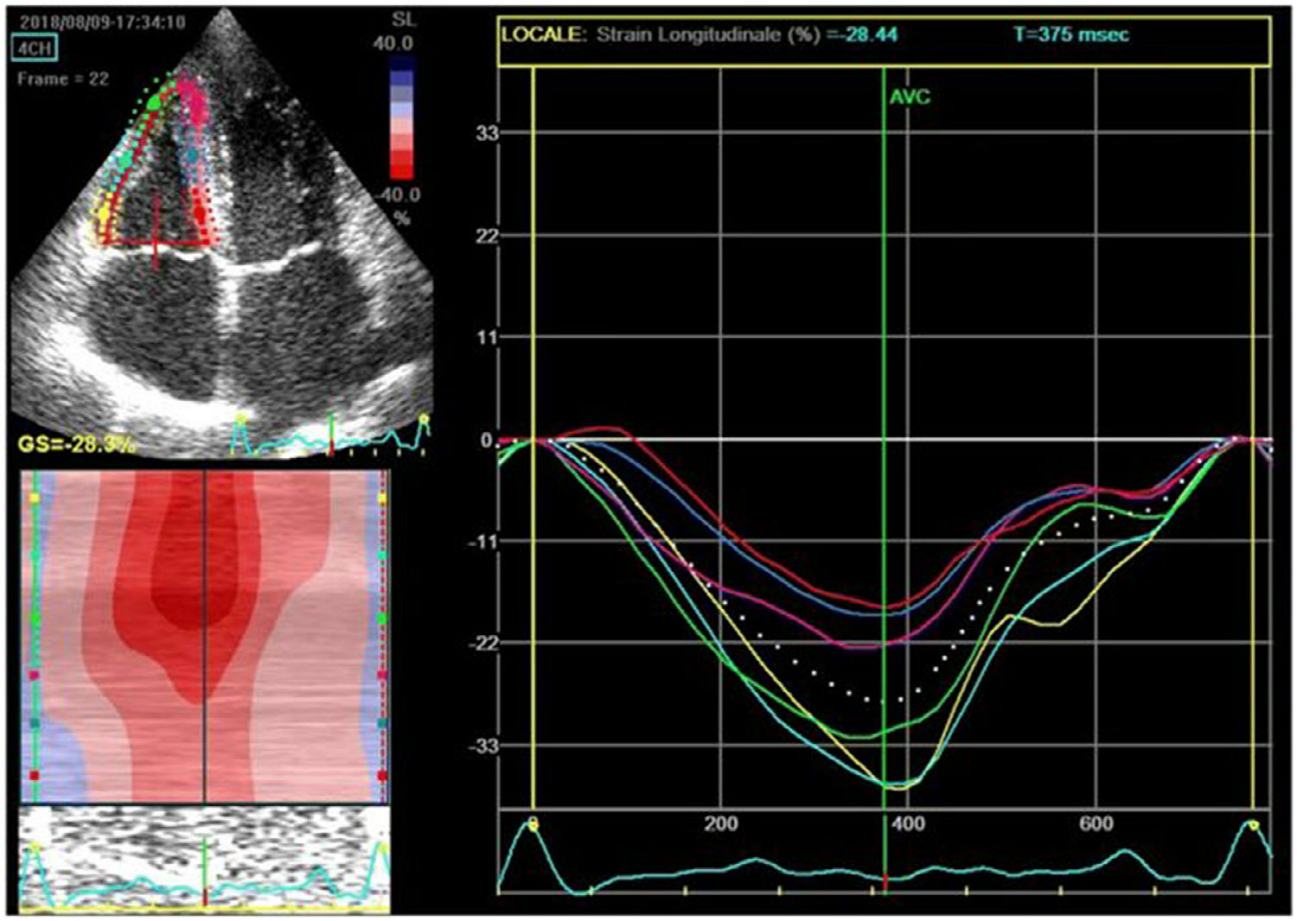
Global right ventricular longitudinal strain (RVLS) Measurement using speckle‐tracking echocardiography analysis.
